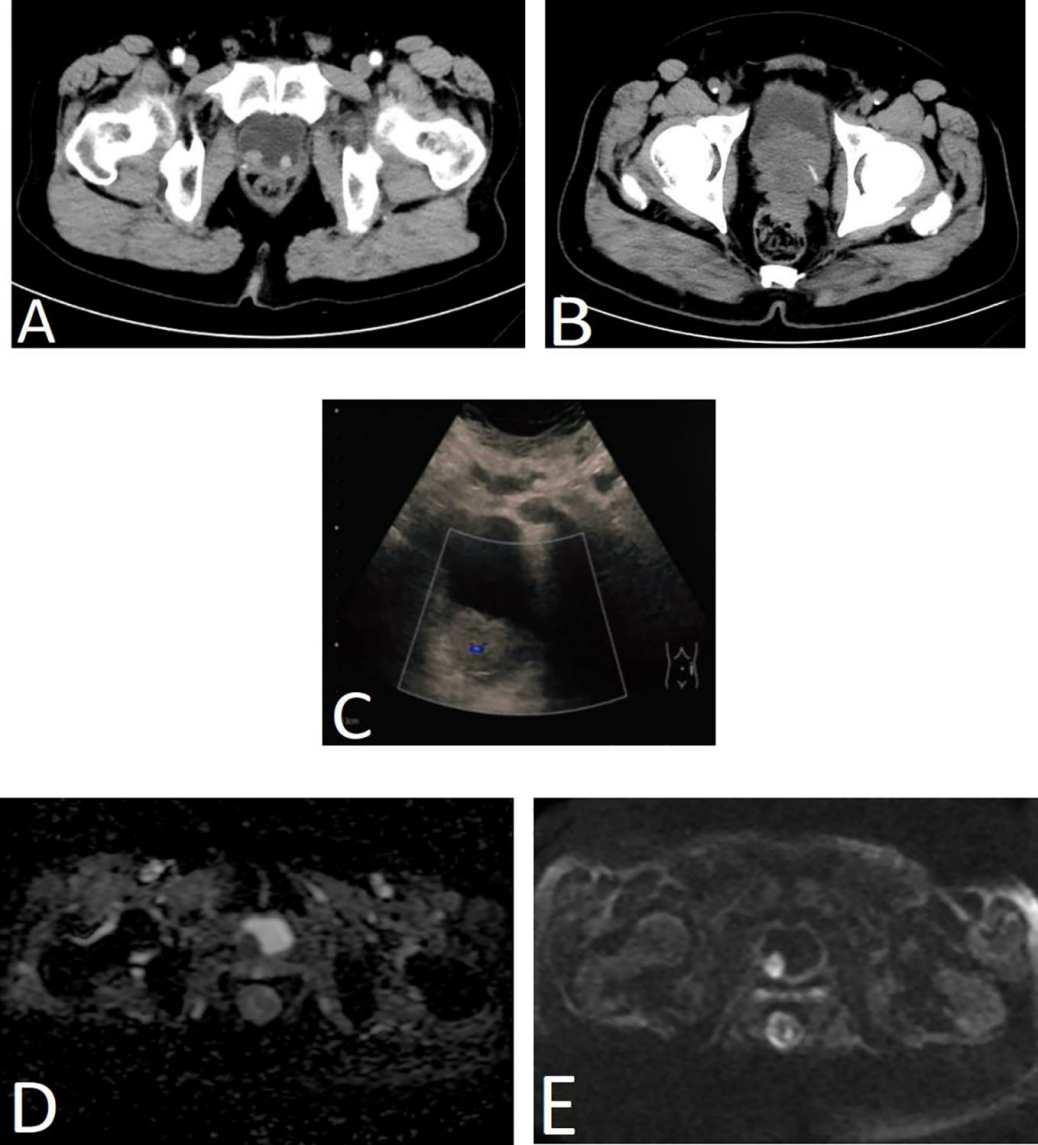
Imaging results of four patients. (A) Enhanced CT scan of the urinary system in case 1. Two nodule-like lesions were detected in the bladder triangle protruding into the bladder, with the right side being larger, measuring approximately 1.6 cm × 1.3 cm in diameter. (B) CT scan of the urinary system in case 2. Multiple hypoechoic nodules were seen in the bladder and near the bladder neck with bladder wall thickening, which was considered as recurrent prostate cancer and bladder metastasis. (C) Bladder ultrasound of case 3. A moderately echogenic lesion of approximately 3.9 cm × 3.3cm in size was seen in the right posterior wall of the bladder with a significant blood flow signal. (D, E) Prostate and bladder MRI of case 4. MRI revealed a lesion in the right posterior wall of the bladder with strong signal in DWI (E) and reduced ADC value (D), and tumor recurrence was considered.
